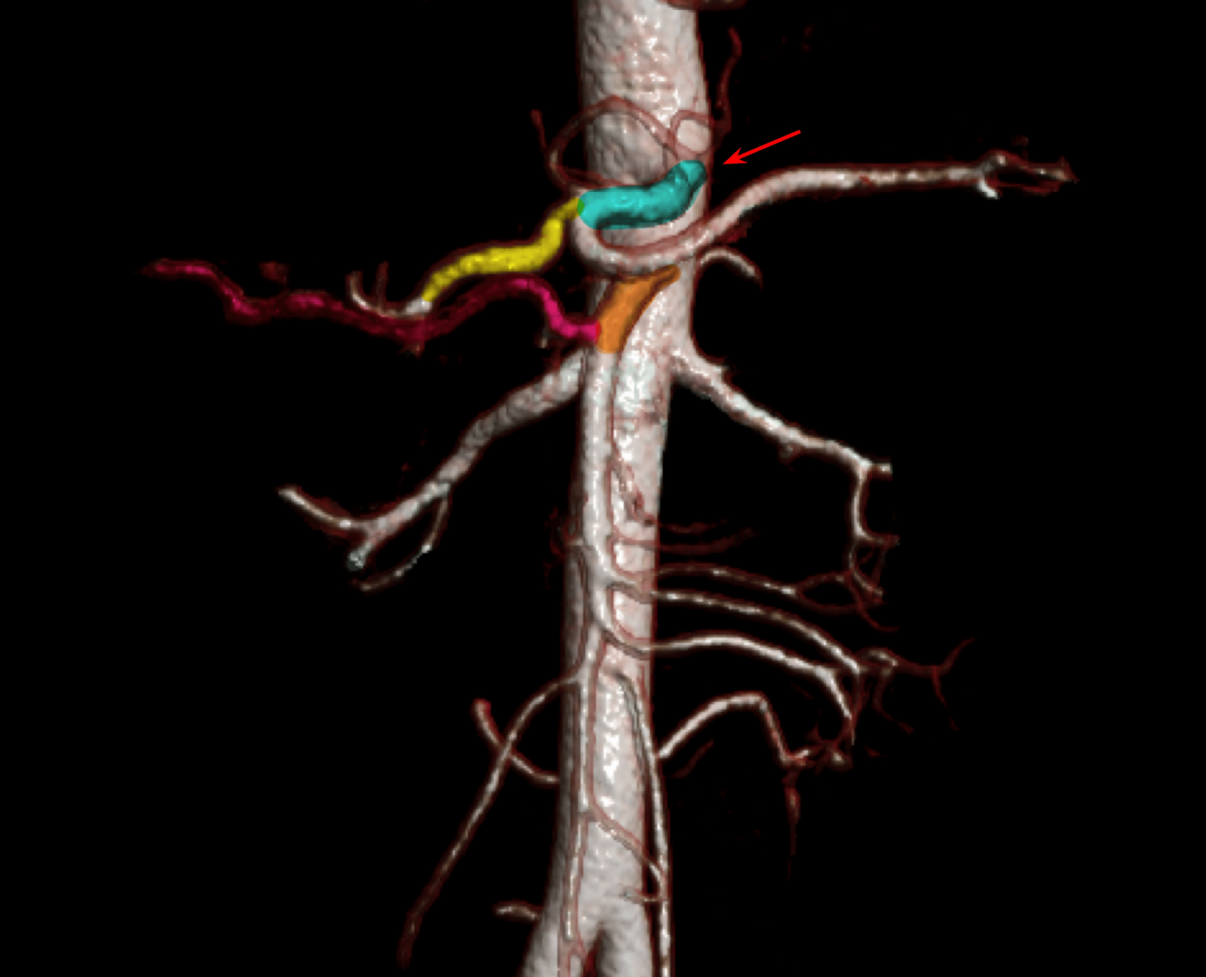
Right hepatic artery replacement. Three-dimensional reconstruction of the upper abdominal contrasted MRI shows that the right hepatic artery (pink artery) is a replacement for the SMA (orange artery). Filling defect in the dorsal region of the celiac trunk caused by extrinsic compression by the middle arcuate ligament (red arrow). The rest of the anatomy shows no abnormalities, with the celiac trunk (blue artery) giving the branch of the common hepatic artery (yellow artery) and its subsequent branches. SMA, superior mesenteric artery
